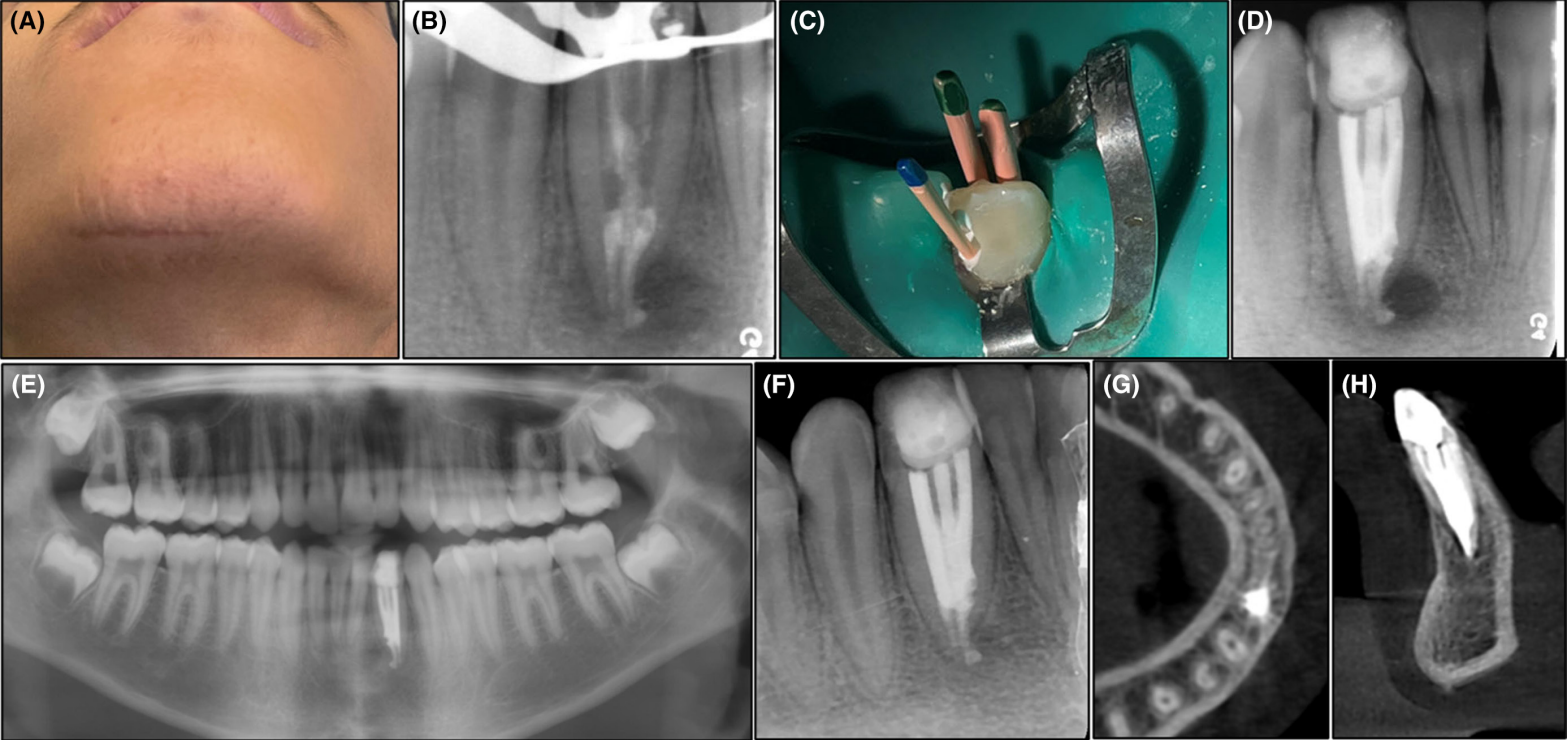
(A) Swelling of the chin and the fistula were resolved. (B) Apical plug with MTA. (C, D) Obturation the canals by EndoSeal MTA with single con technique. (E) 12‐month follow‐up. (F) Panoramic radiograph after 24‐months shown complete bone heling. (G, H) CBCT revealed Complete healing of the periradicular lesion and formation of a bone cortex.
